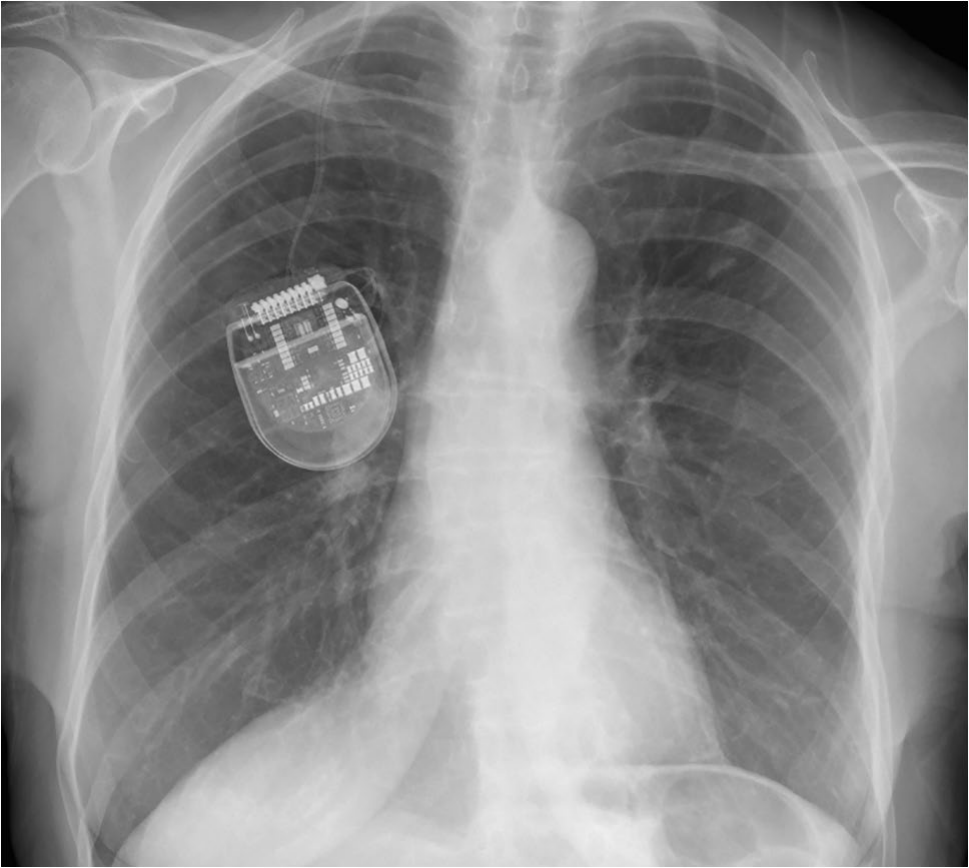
Postoperative chest x-ray with no signs of twisting of the electrodes
